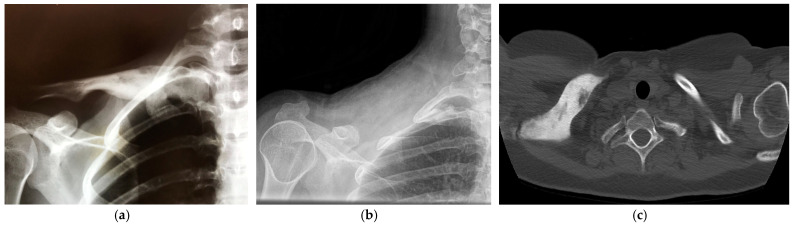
(a) X-ray and CT scan (b) showing diffuse involvement of the clavicle of patient 1 (a) and patient 2 (b) with bone thickening and sclerosis including the sternoclavicular joint. (c) X-ray after clavicular resection.
